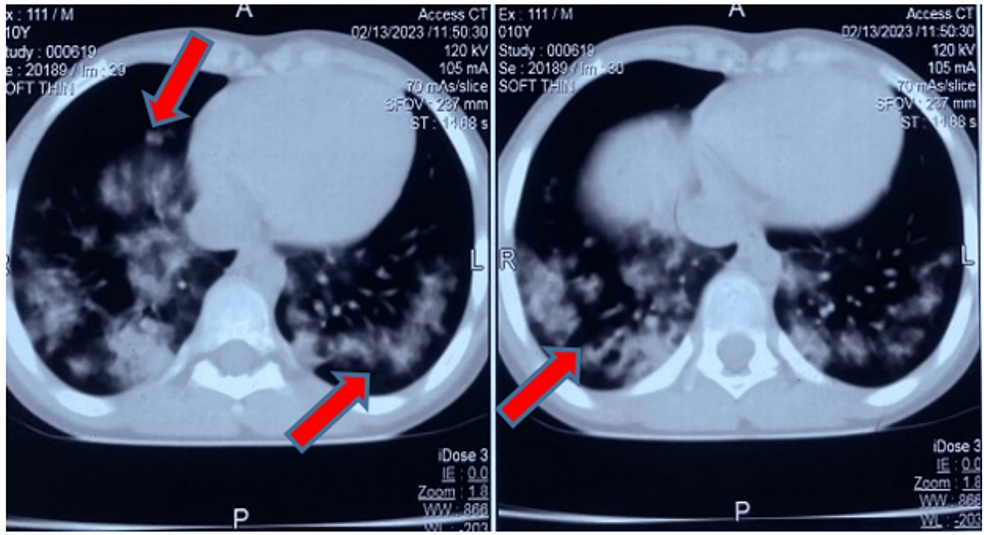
Contrast-enhanced computed tomography of the chest. Red arrows show multifocal confluent hyperdense lesions present in bilateral lower lung segments.
